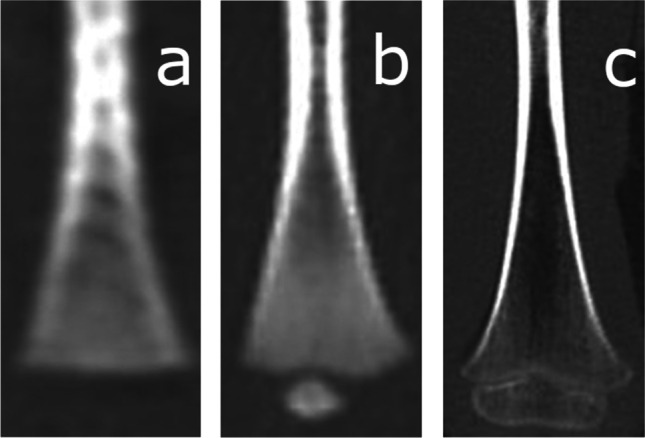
Computed tomography images depicting the development of secondary ossification center in the distal femoral epiphysis: A Stage 1 – no secondary ossification centers; B Stage 2 – the presence of an irregularly shaped secondary center of ossification that occupies less than half of the width of the metaphysis; C Stage 3 – development of ossified epiphysis, initially the epiphyseal plate spans more than half of the width of the metaphysis, and towards the end of development the epiphysis and the metaphysis are of comparable width
